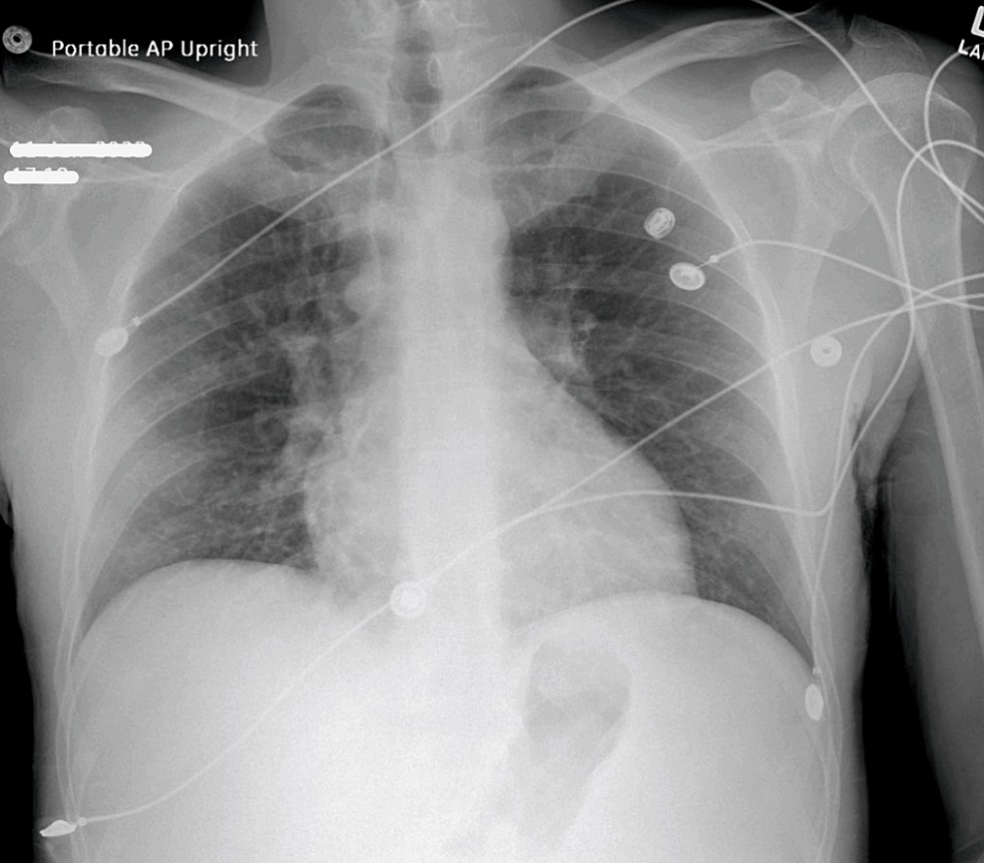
Patient's chest x-ray on admission
